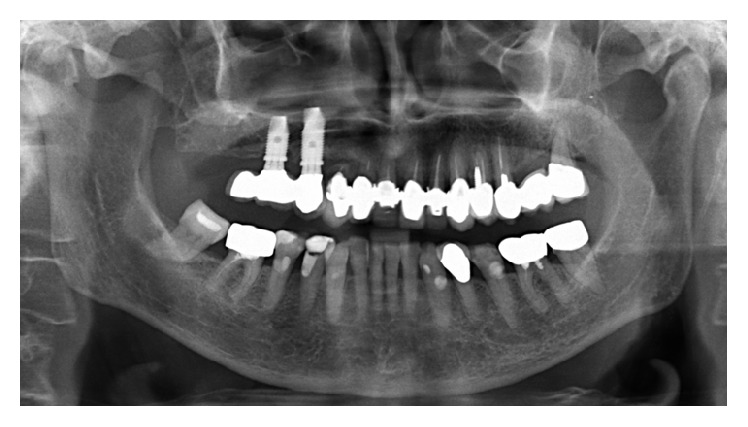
Panoramic X-ray: unilocular, well-defined radiolucency of the right maxillary sinus region without dental focus.
